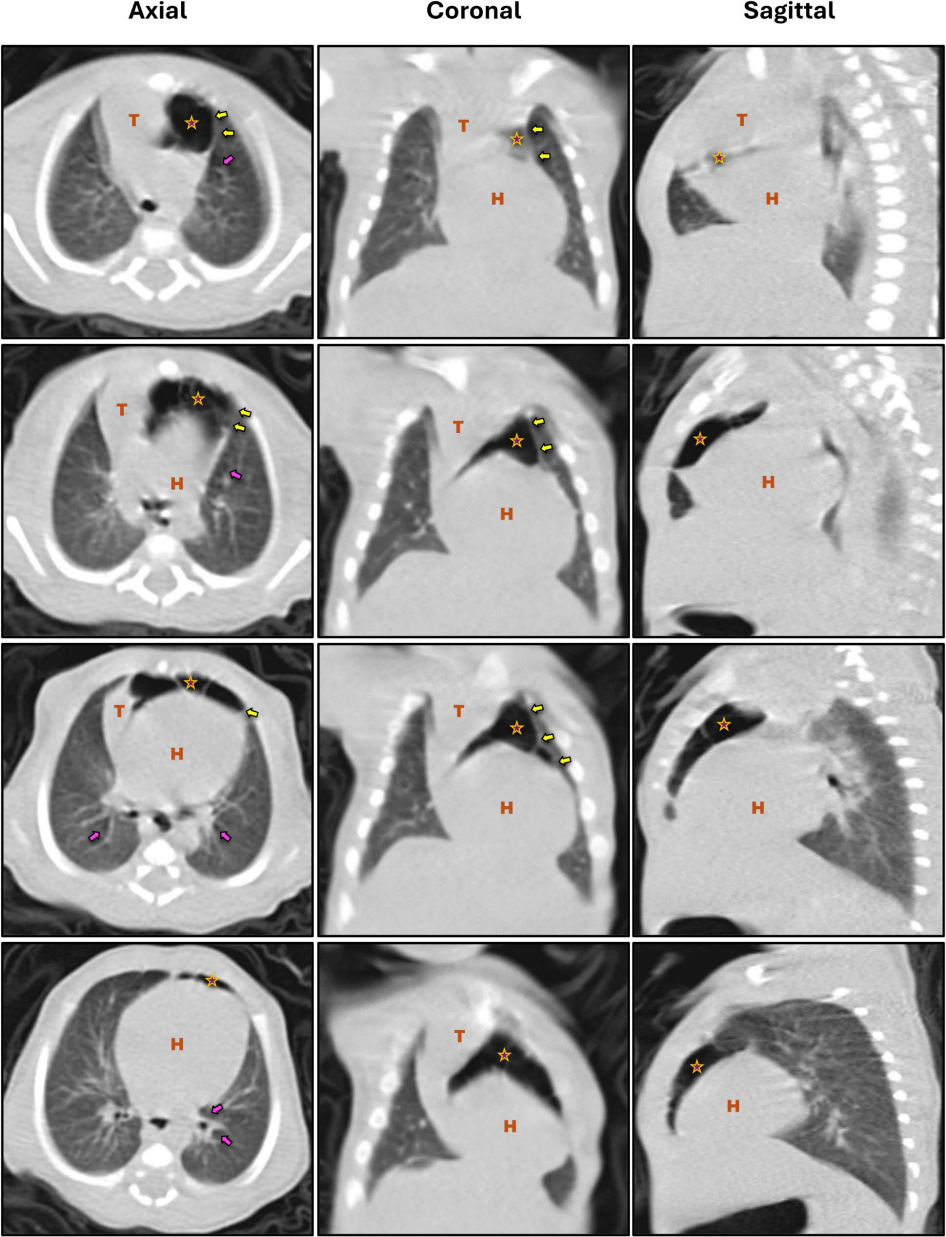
CT of a full-term neonate with SPM (Patient-1). Yellow arrows: the lateral borders of the fascia overlying the thymus and pericardium. Pink arrows: hyperaeration around the main bronchi, indicating air dissection through the peribronchovascular sheaths. The Asterisk (*) multiloculated hyperlucency in the anterior mediastinum displacing the thymus superolaterally, with thin radiopaque lines showing the hyperinflated thymic capsule. (T) Thymus; (H) Heart. All segmental bronchi are within lungs, and no lobar emphysema is noted
